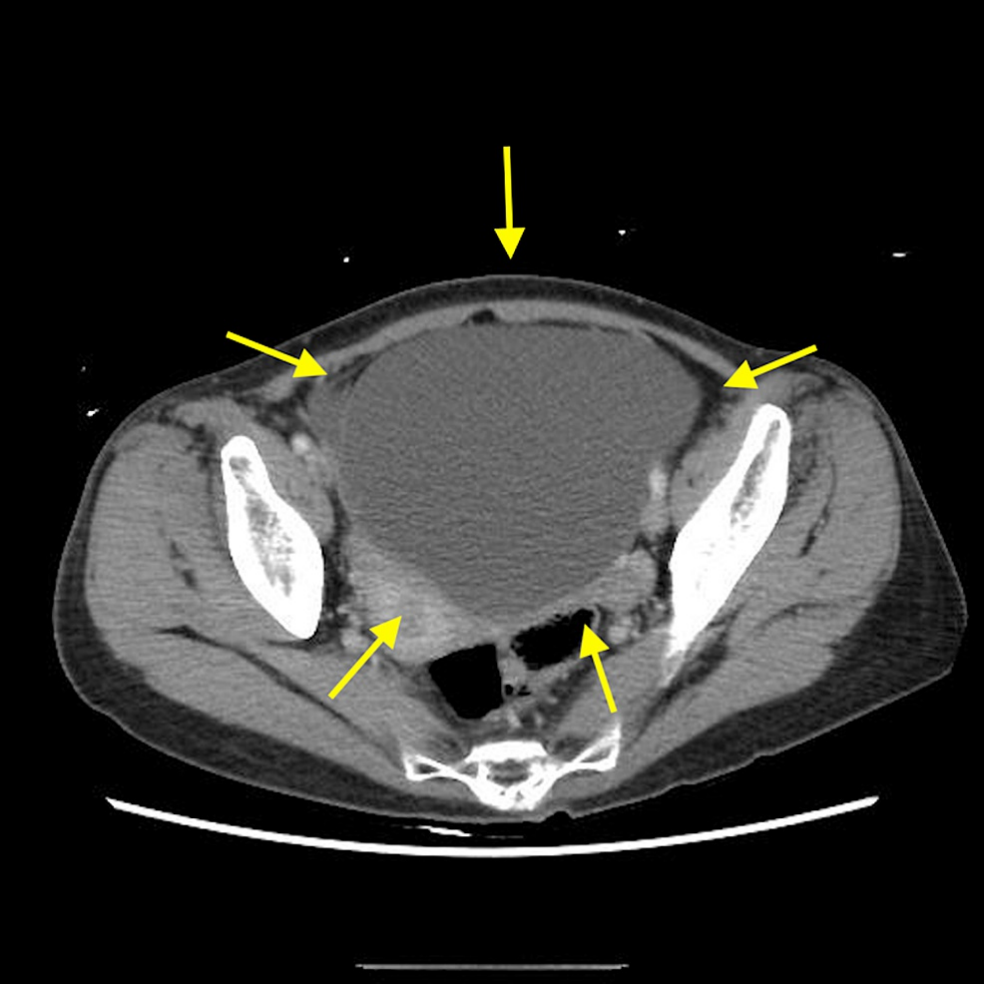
Computed tomography (CT) of the ovarian tumor Abdominal computed tomography showing a 10-cm-large monoblastic ovarian tumor Yellow arrows: ovarian tumor
